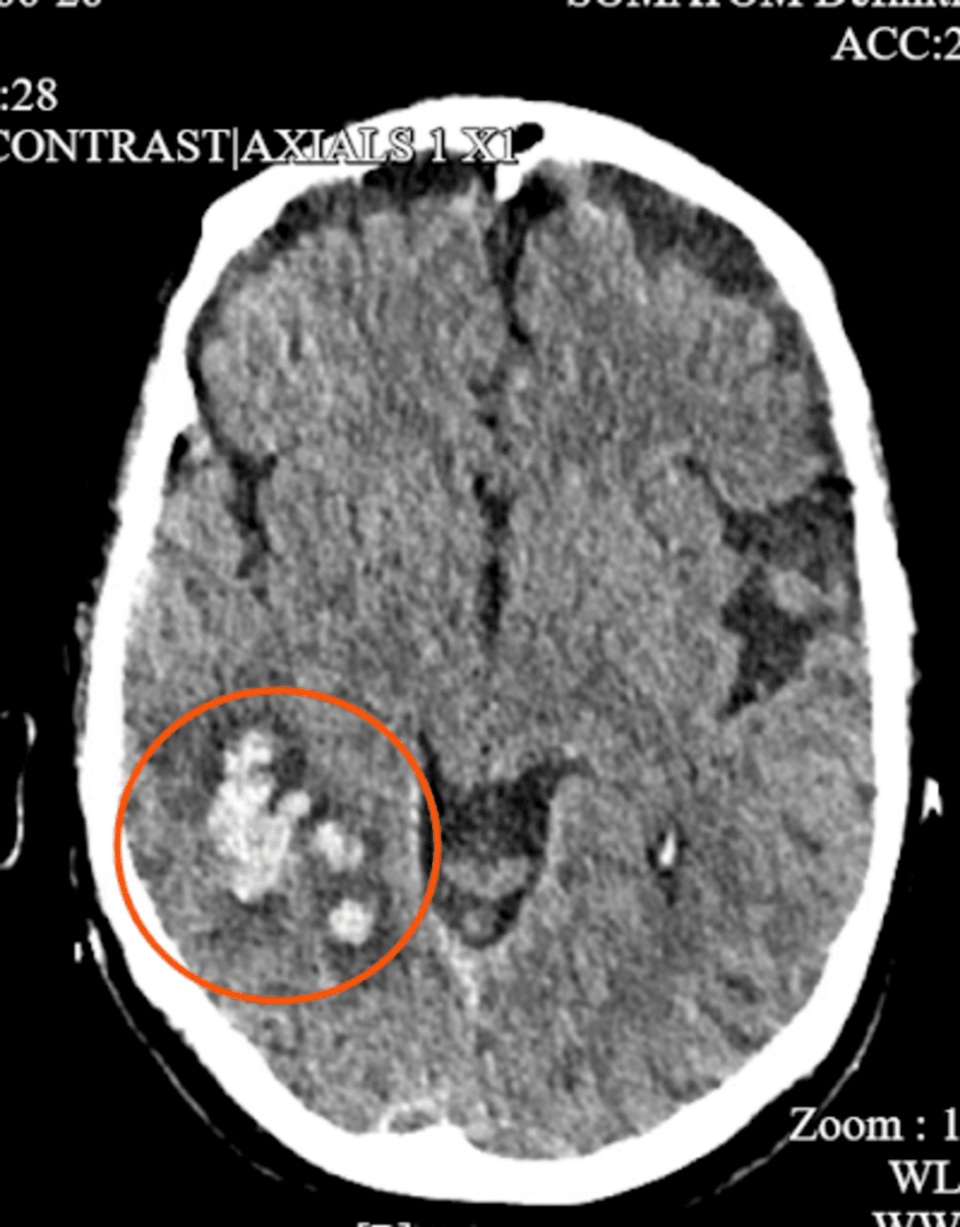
CT Head without contrast demonstrates an acute ICH at the posterior right temporal lobe, partially extending into the parietal lobe, with adjacent edema, measuring approximately 2.27 cm in width CT: Computed tomography scan; ICH: Intracerebral hemorrhage
